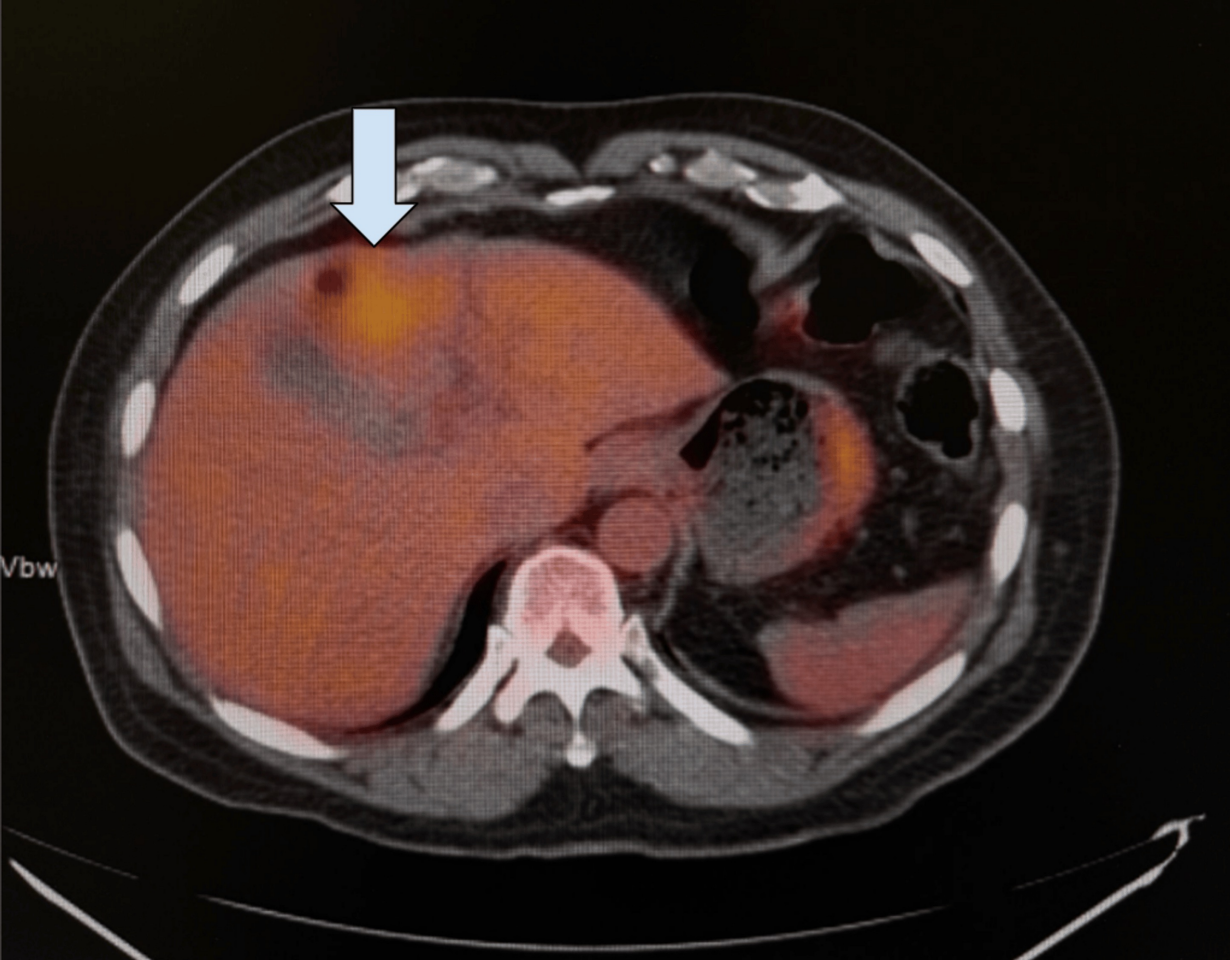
PET scan demonstrating focal increased uptake in the inferior segment of the liver, close to the hepatic flexure, with an SUV of 5.8 (arrow). SUV: standardized uptake value
